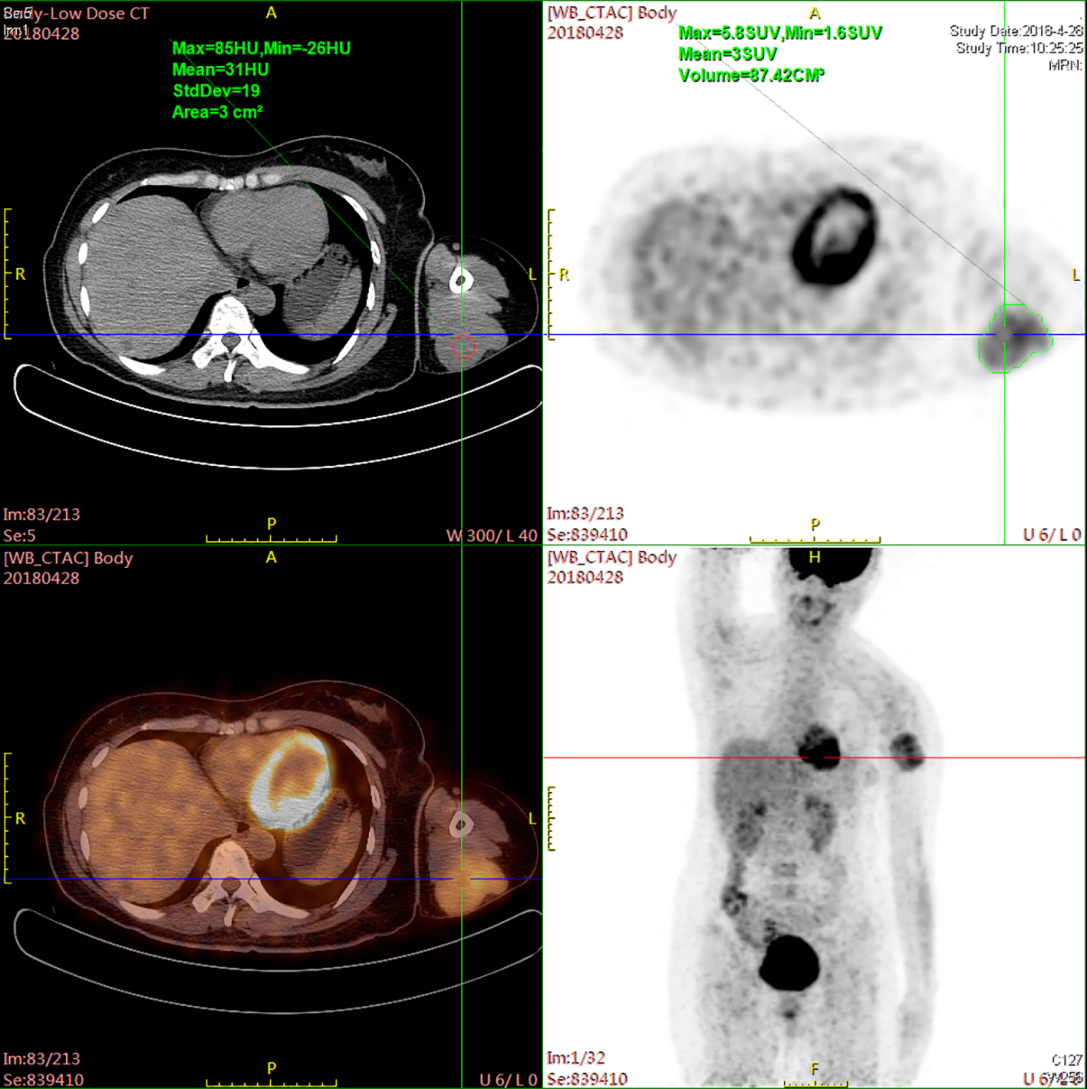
PET-CT scan revealing increased metabolic activity within a nodular soft tissue density located in the posterior subcutaneous area of the upper arm.
